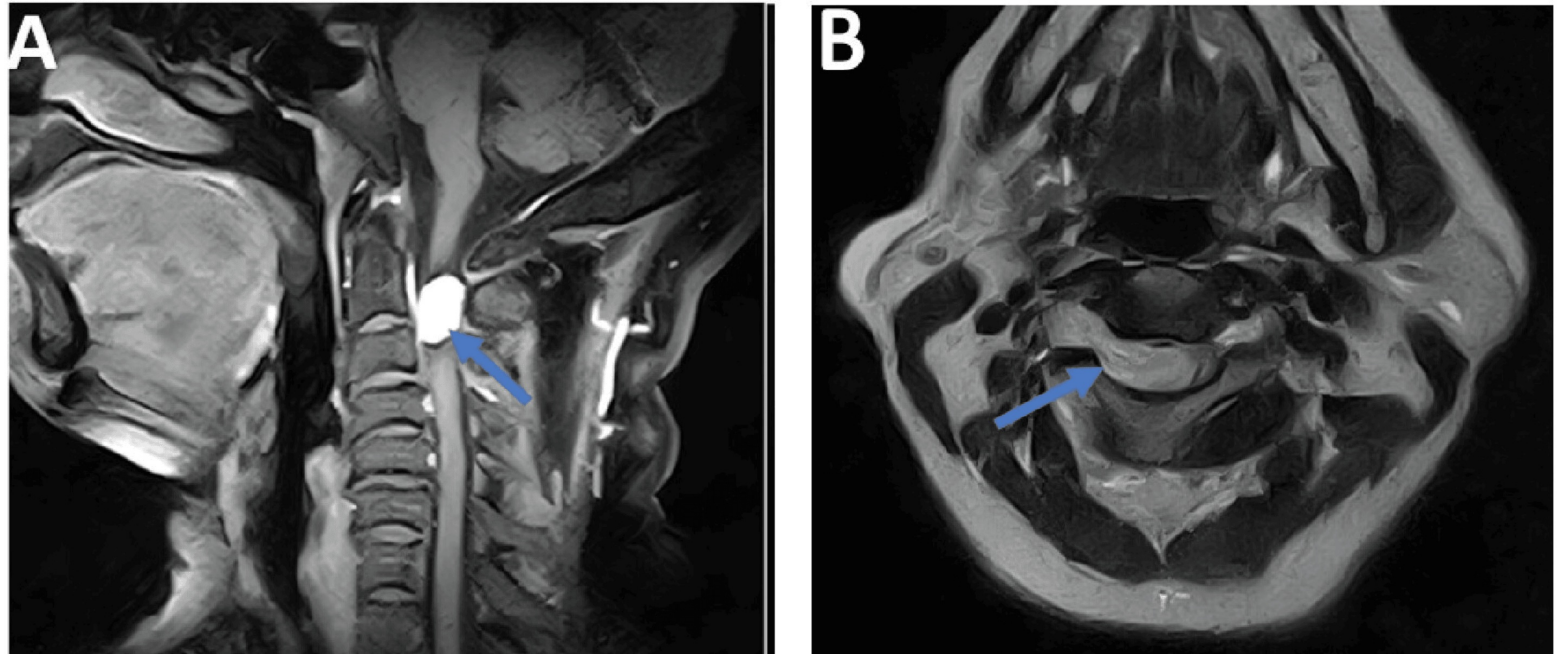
(A) Sagittal T1-weighted MRI image showing a contrast-enhancing intradural mass at the C2–C3 level (blue arrow); (B) Axial T1-weighted MRI image showing a right anterolateral intradural extramedullary mass in contact with the C2 nerve root (blue arrow).
